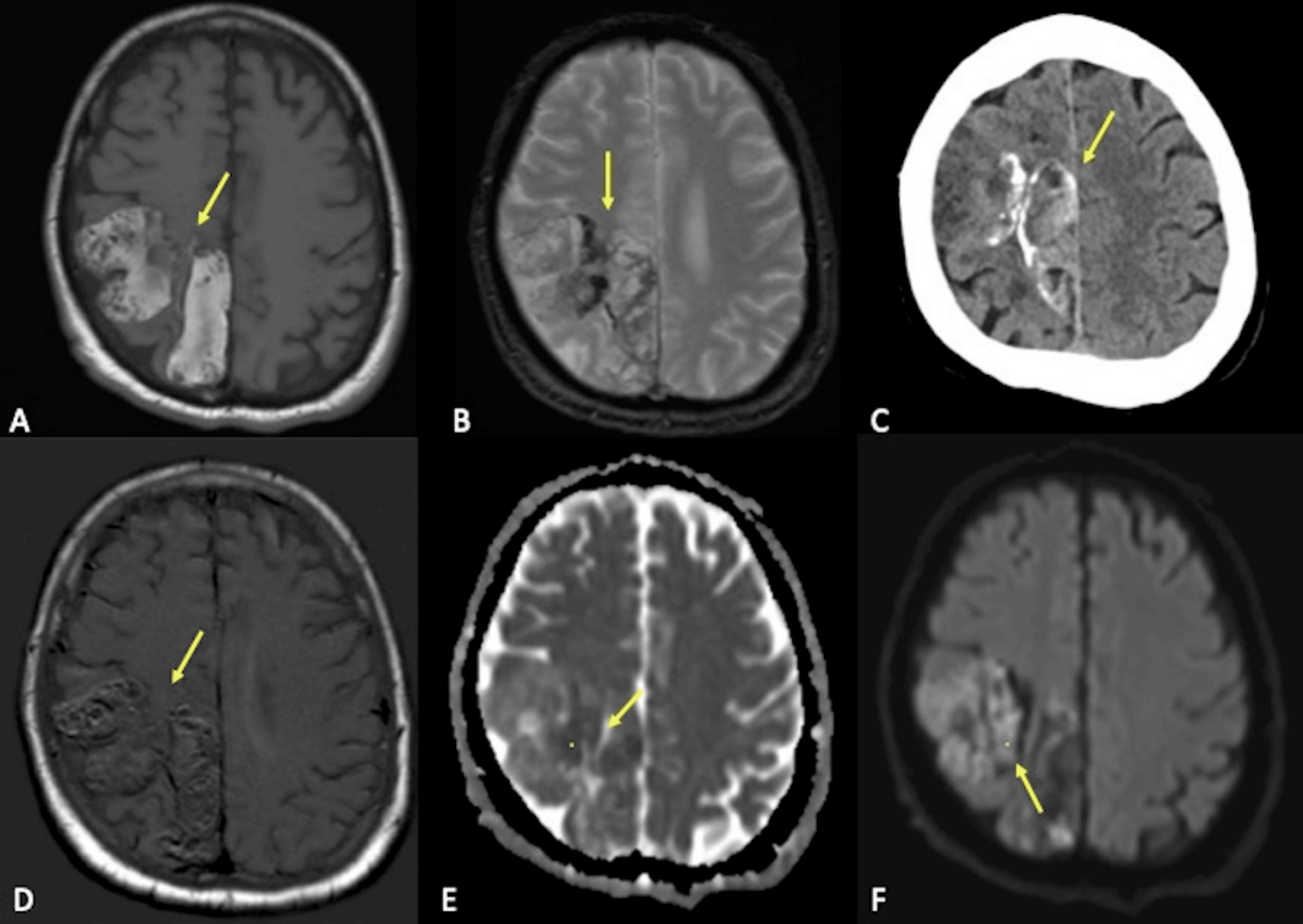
Axial CT and MRI sequences A: An axial T1-weighted sequence at the level of the centrum semiovale demonstrates a heterogeneous but predominantly T1 hyperintense lesion in the right frontoparietal region. B: An axial gradient echo sequence shows paramagnetic susceptibility within the lesion, correlating with calcification on CT. C: An axial non-contrast CT scan depicts the right frontoparietal lesion containing calcifications and internal areas of hyperattenuation, corresponding to T1 hyperintensity on MRI, suggestive of proteinaceous contents. D: An axial T1 post-contrast subtraction sequence shows a lack of enhancement in the right frontoparietal lesion. E and F: Axial diffusion and ADC show limited areas of restricted diffusion (yellow dot) in the right frontoparietal lesion.
